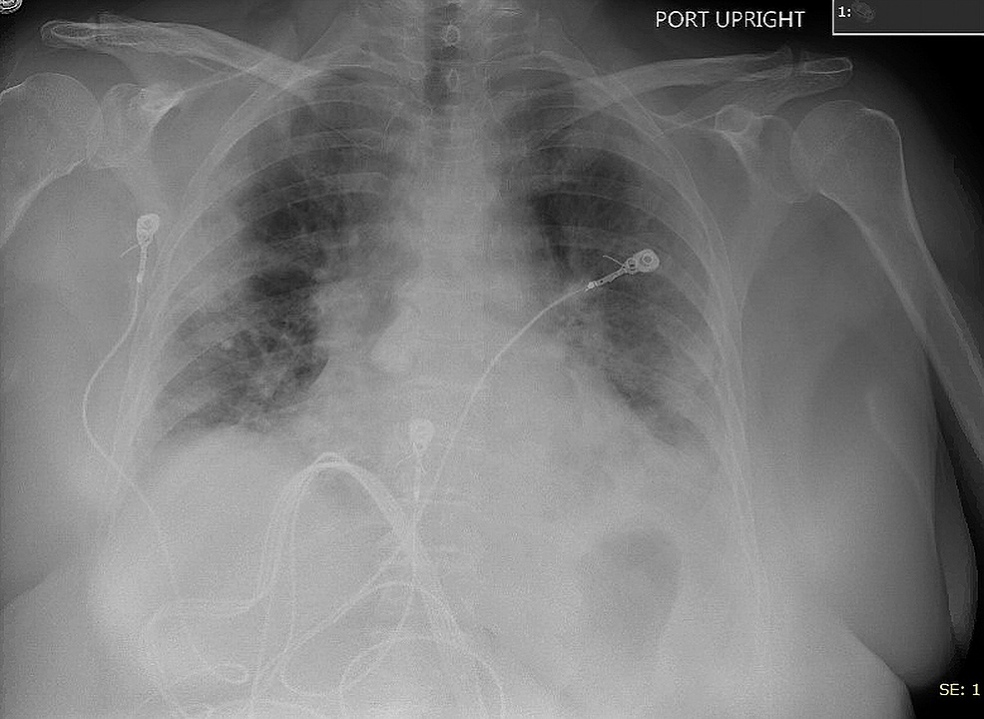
Admission chest X-ray showing bilateral peripheral infiltrates
